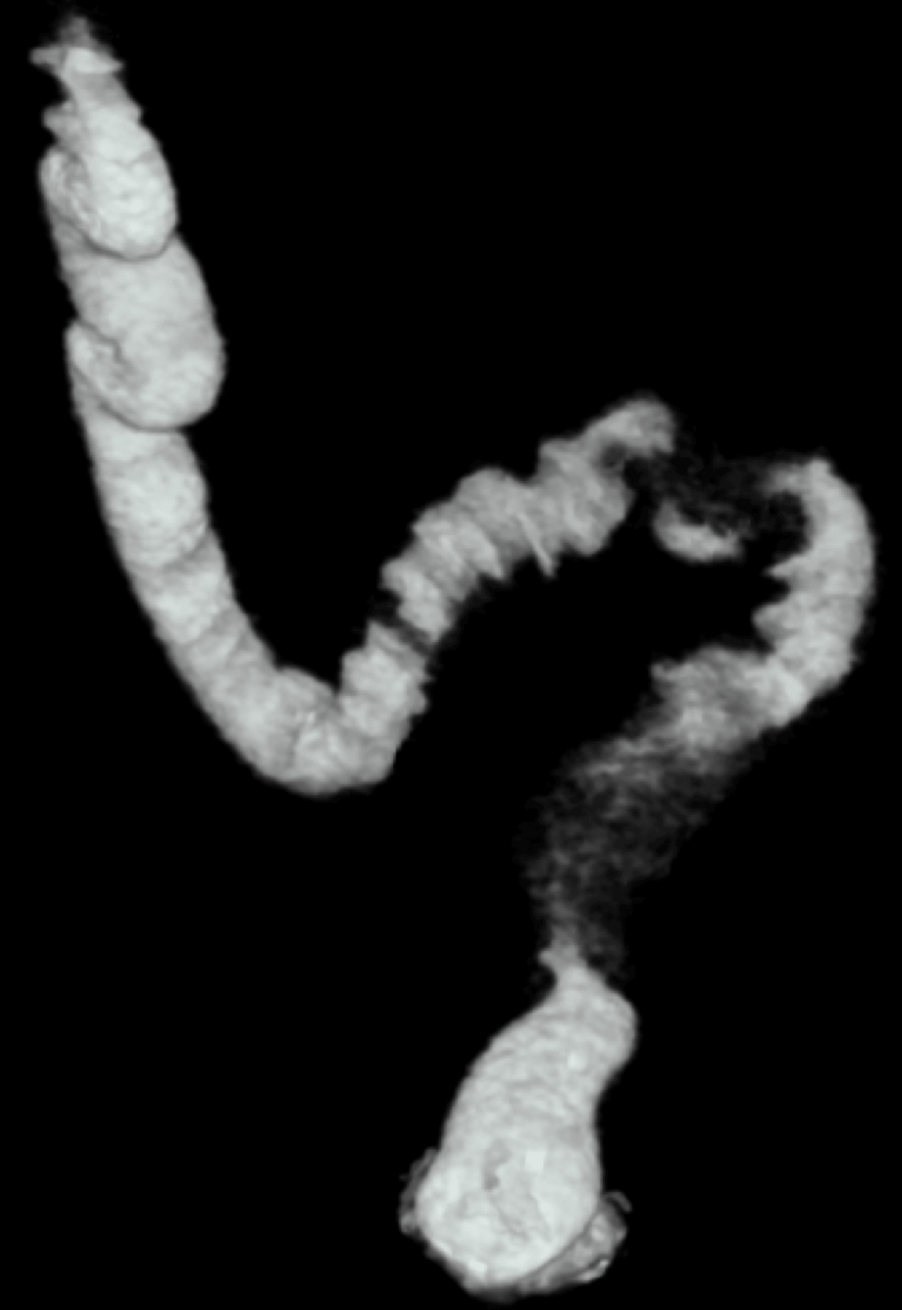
3D reconstruction of the contrast-enhanced abdominopelvic CT scan It shows dilation of proximal colonic loops and a constrictive ring in the sigmoid colon with fibrosed peritoneal tissue.
